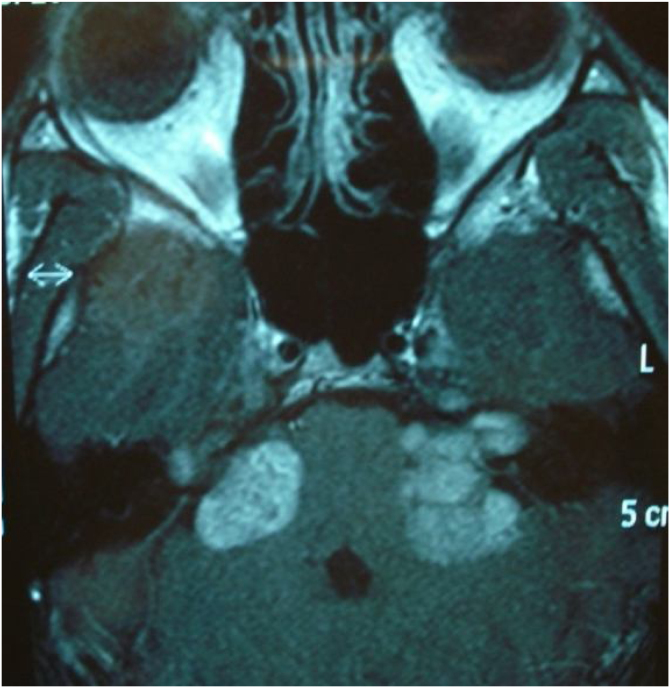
Magnetic resonance imaging (contrast-enhanced T1-weighted image). Axial plane. Presence of bilateral vestibular schwannoma (neurofibromatosis type 2).
